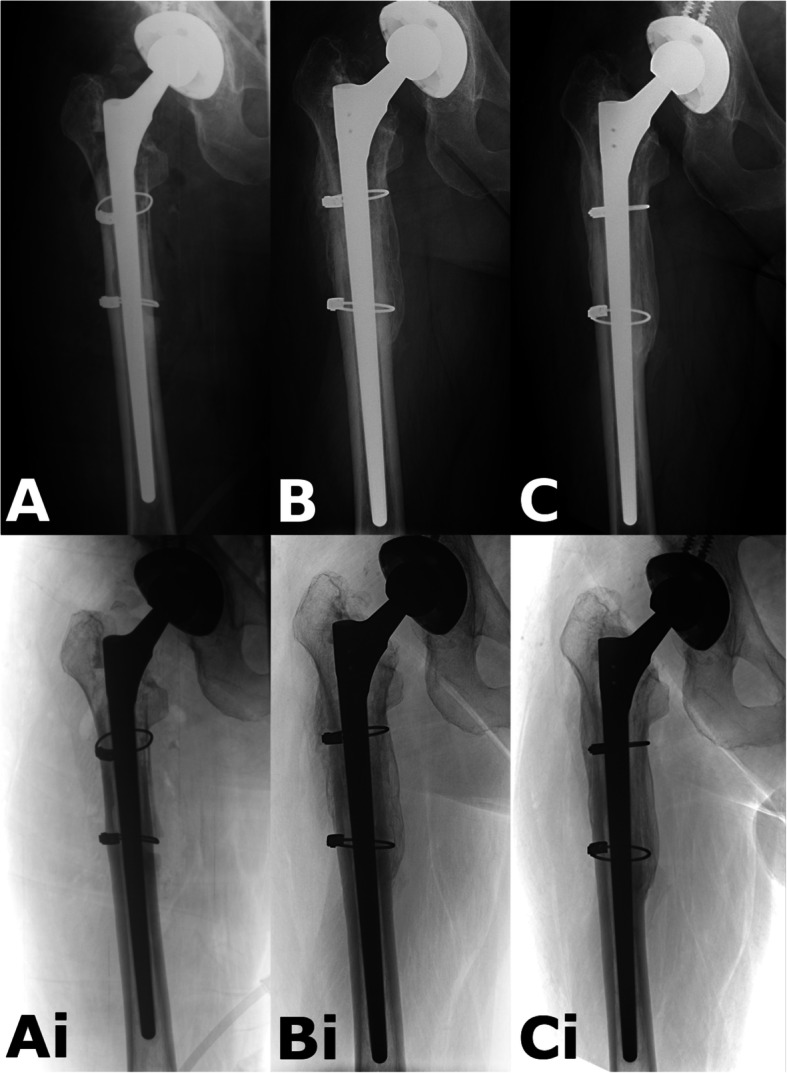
Example of a PFF case. a: Immediate postop radiograph after revision. The poor quality of the proximal bone stock is unequivocal. b: obvious new bone formation seen at 6 month follow-up. There is some subsidence. c: Radiograph 2 years after the operation. Complete reconstitution of bone stock. Ai-Bi-Ci: Shows the same pictures in inverted view (Patient 07)
